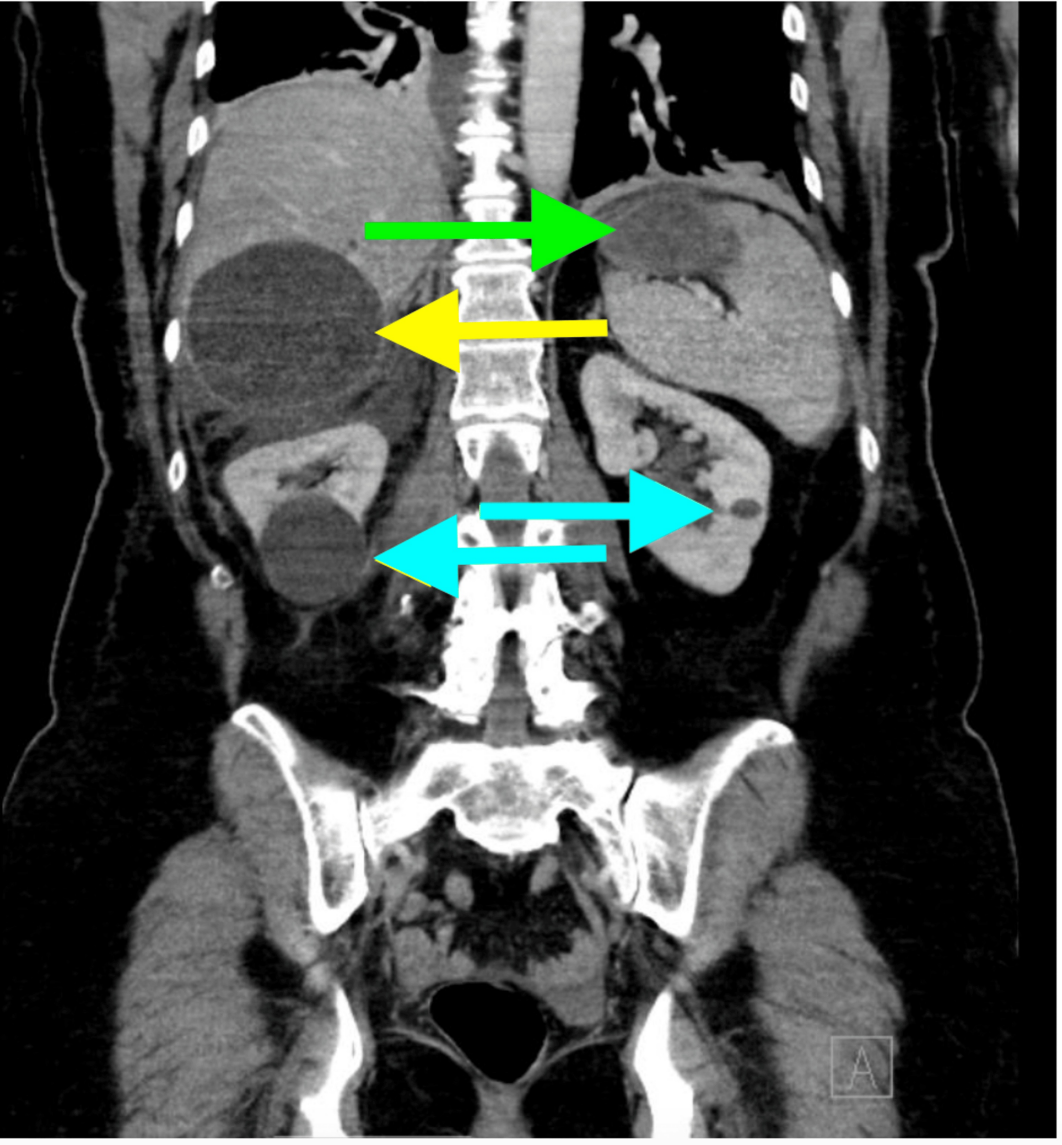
Splenic, hepatic, and renal cysts indicated by green, yellow, and blue arrows, respectively, on CT scan CT: computed tomography
